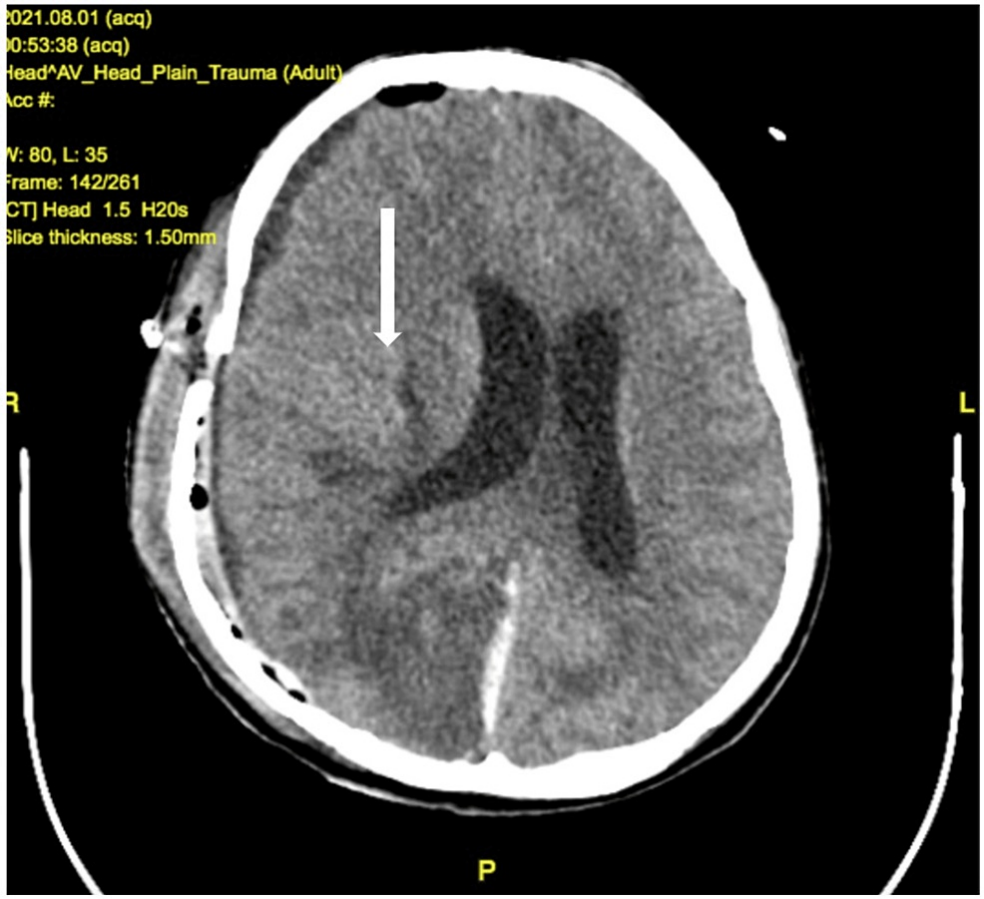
Postoperative CT brain showing diffuse cerebral edema with a significant decrease in the hematoma.
